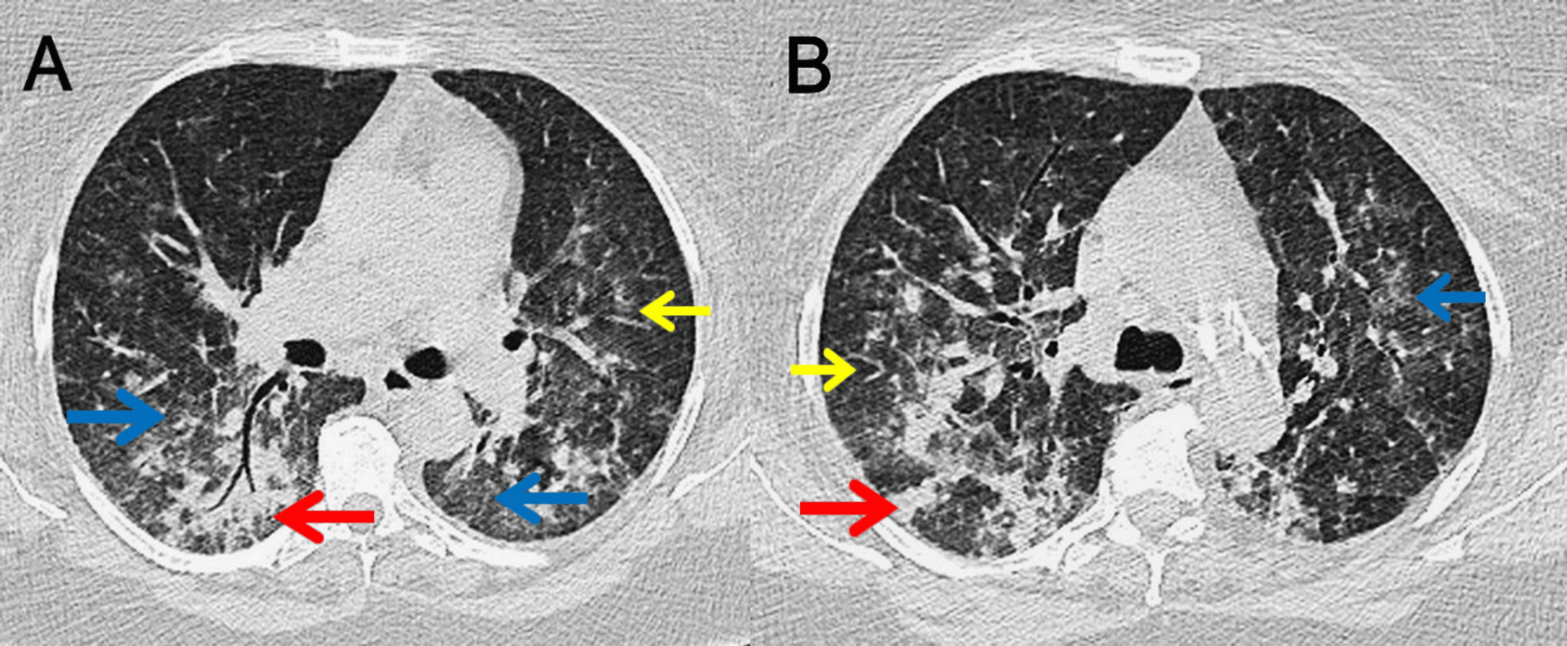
HRCT of the thorax on Day 1 A (basal): Lower-lobe predominant dependent posterior consolidation on diffuse bilateral GGO with mild interlobular septal thickening; no pleural effusion or traction bronchiectasis. B (mid-thoracic): Patchy peripheral/peribronchovascular GGO with subtle reticulation; less consolidation than the bases. The arrows identify key abnormalities: red highlights areas of consolidation, blue marks GGO, and yellow indicates interlobular septal thickening. Overall pattern, in context, supports ARDS. GGO: ground-glass opacities, ARDS: acute respiratory distress syndrome
